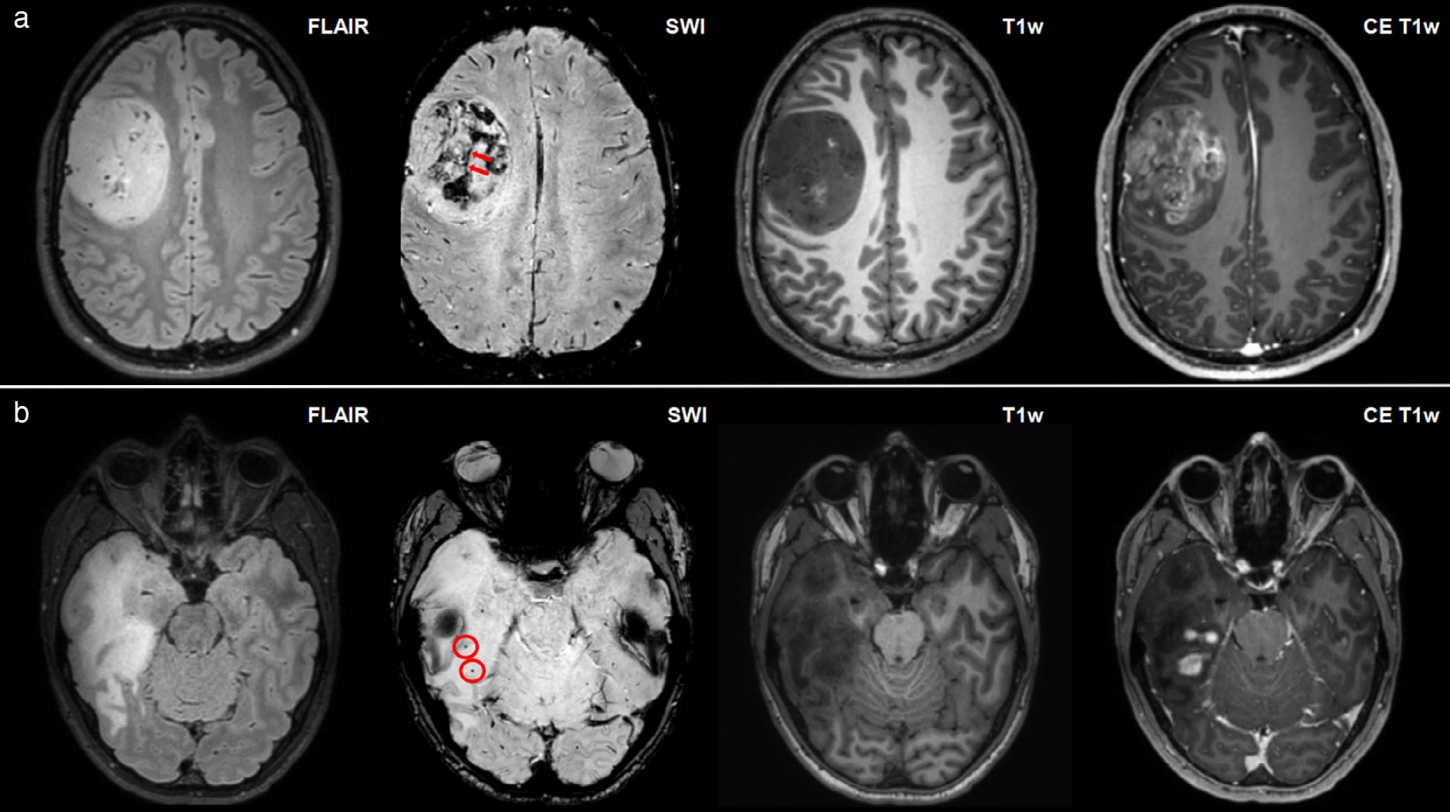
Panel (a) depicts a right frontal glioblastoma in a 40‐year‐old male and panel (B) depicts a right temporal anaplastic astrocytoma in a 32‐year‐old female, with high signal on fluid‐attenuated inversion recovery (FLAIR), low signal on T1‐weighted imaging, and partial contrast enhancement according to contrast‐enhanced T1‐weighted imaging. Intratumoral susceptibility signal (ITSS) abnormalities can be found according to susceptibility‐weighted imaging (SWI) in both patient cases (a: multiple dot‐like and fine linear ITSS abnormalities corresponding to ITSS grade 3, red arrowheads point at a prominent linear ITSS within the lesion; b: only few dot‐like ITSS abnormalities corresponding to ITSS grade 1, red circles enclose two exemplary dot‐like signal drops within the lesion).
